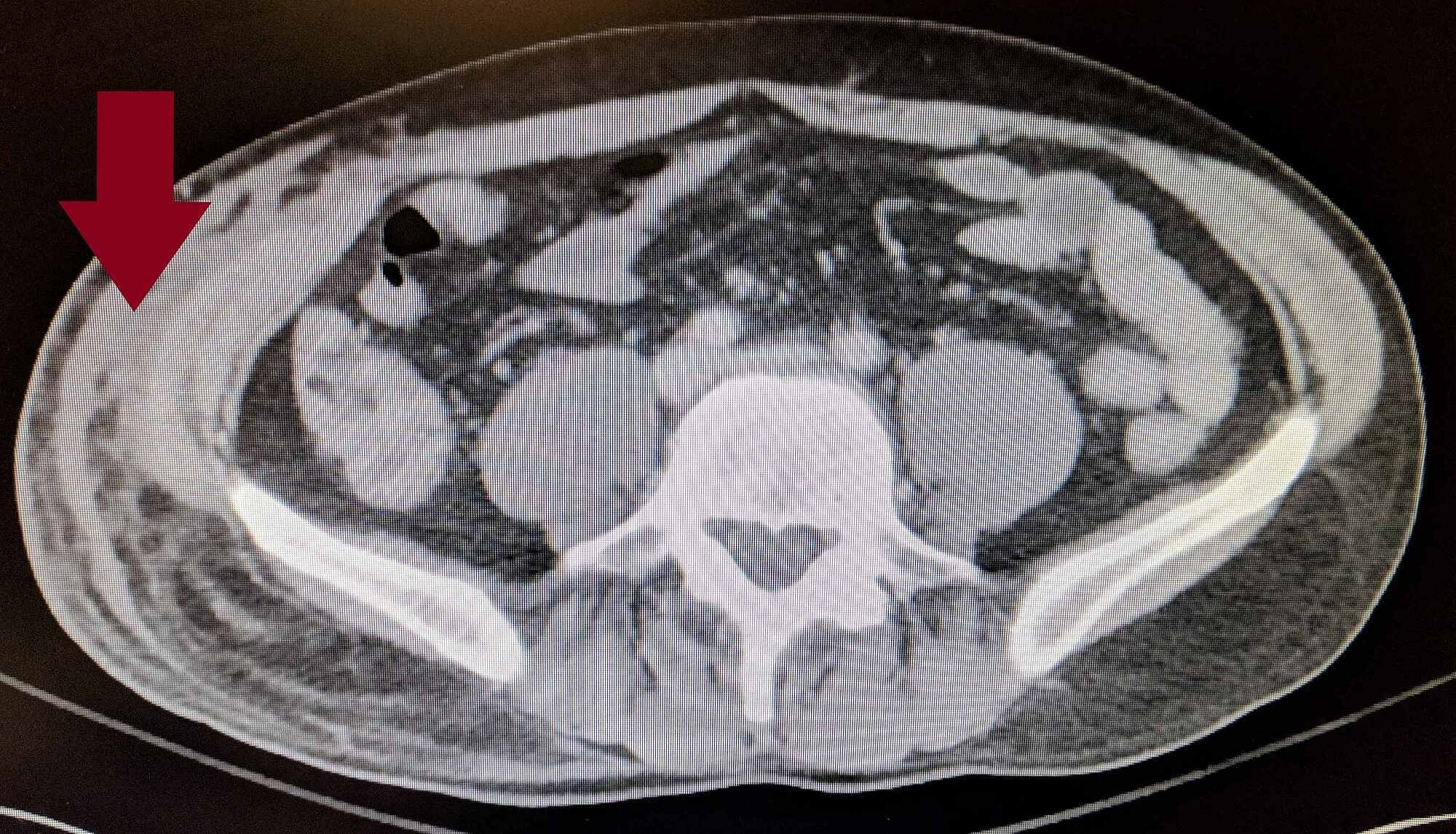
CT chest, abdomen, pelvis revealing collection of fluid in the fascial planes in the pelvic region.
